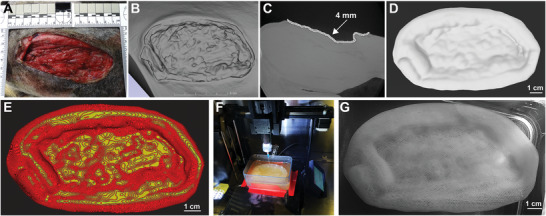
Fabrication of FRESH printed dECM patches. A) Photograph of the VML wound. B) CT scan of the wound bed. Using image segmentation, a 4 mm uniform thickness patch was created from the geometry of the wound bed. C) Side view of a slice of the CT scan of the VML wound with patch model to visualize the depth of the wound and thickness of patch. D) The patch model was cropped around the wound bed and exported as an STL. E) The CAD model of the patch was imported into Ultimaker Cura to generate the print path and create a model with 40% infill. F) FRESH 3D printed ECM hydrogel patch made of 1:1 ratio of dECM and collagen type I bioink. G) Photograph of 3D printed patch submerged in 50 mM HEPES.
